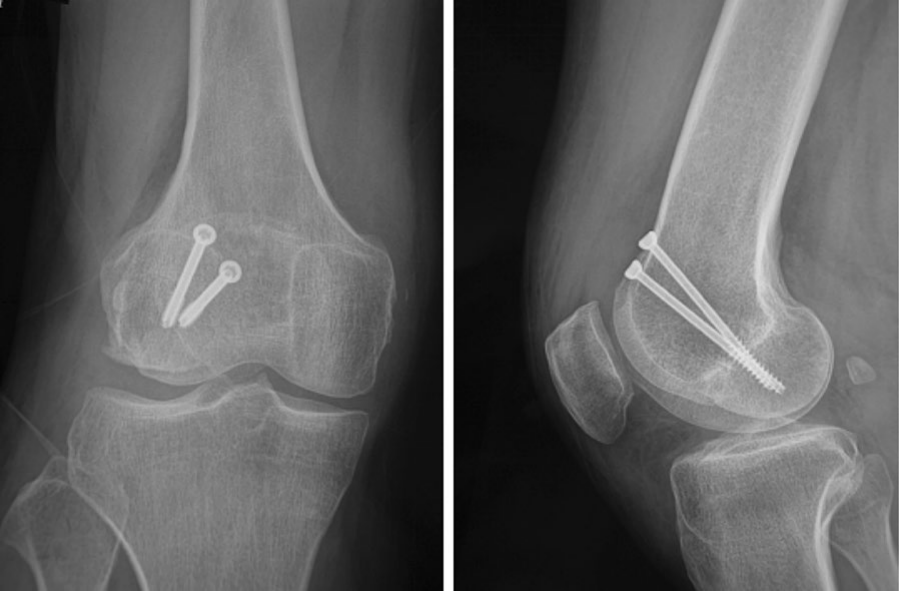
Anteroposterior and lateral radiograph shows the fracture is fixed with two anteroposterior parallel screws
